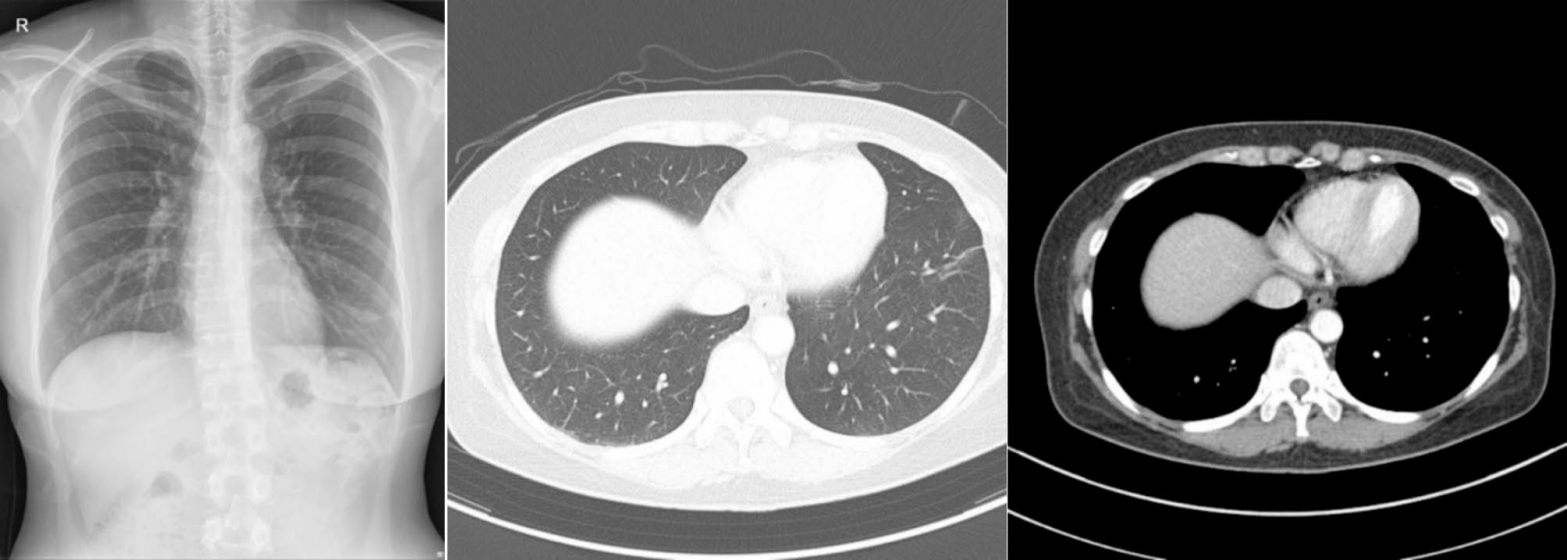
(a), (b), (c) Chest x-ray photograph and chest CT showing the absence of the peripheral pulmonary nodule
